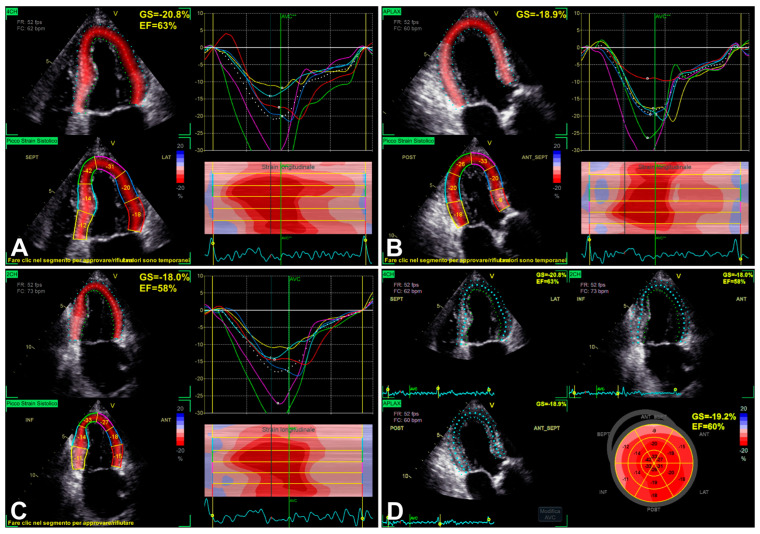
An example of LV function evaluation by LV GLS, in the presence of SAS, assessed from 2D apical four-chamber view (A), three-chamber view (B), two-chamber view (C), and finally reported “bull’s eye” GLS plot (D). Note: the most impaired longitudinal strain results in basal segments (see “bull’s eye” plot in D), showing the typical gradient between basal and apical segments in decreasing longitudinal function [35].
